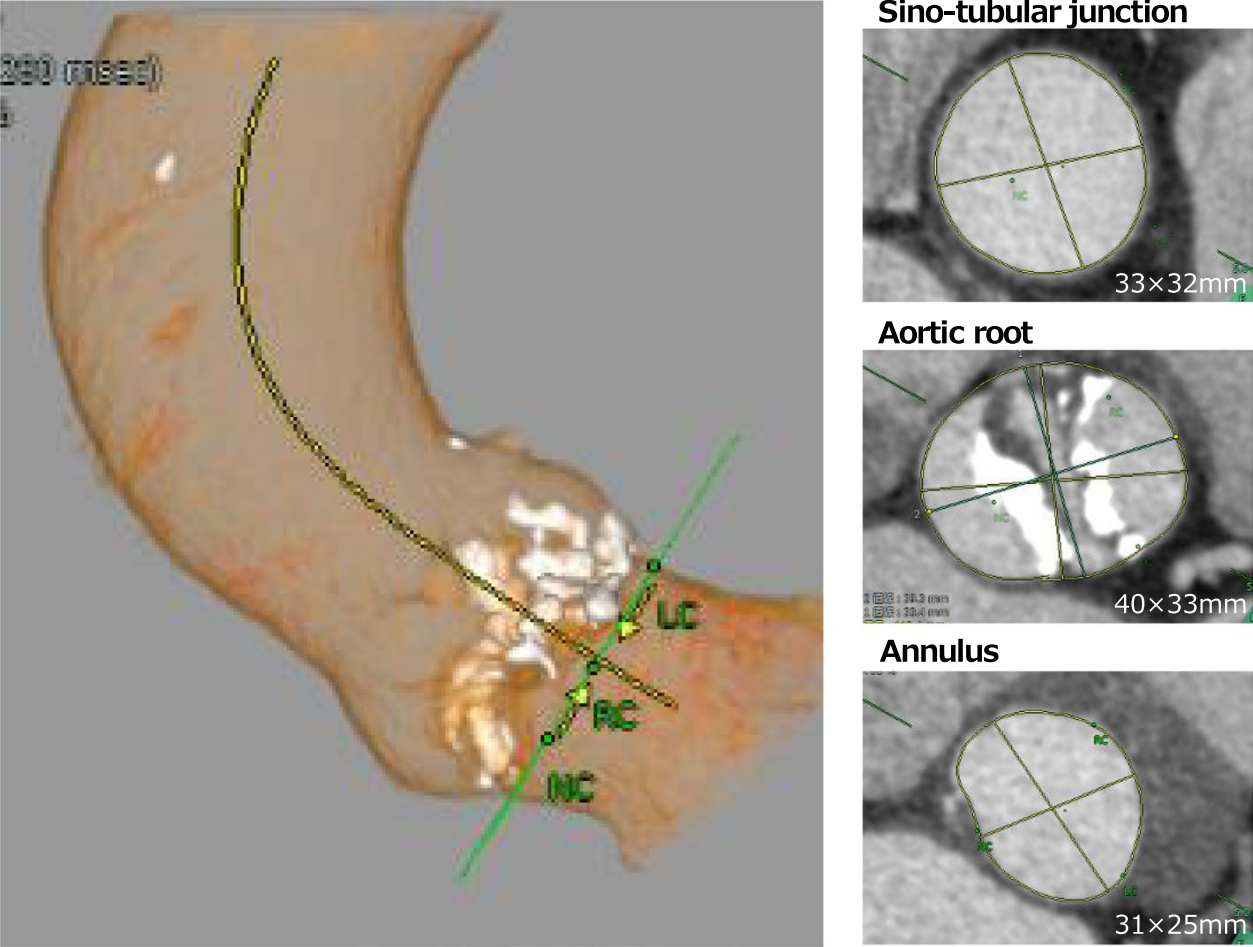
Preoperative computed tomography evaluation
